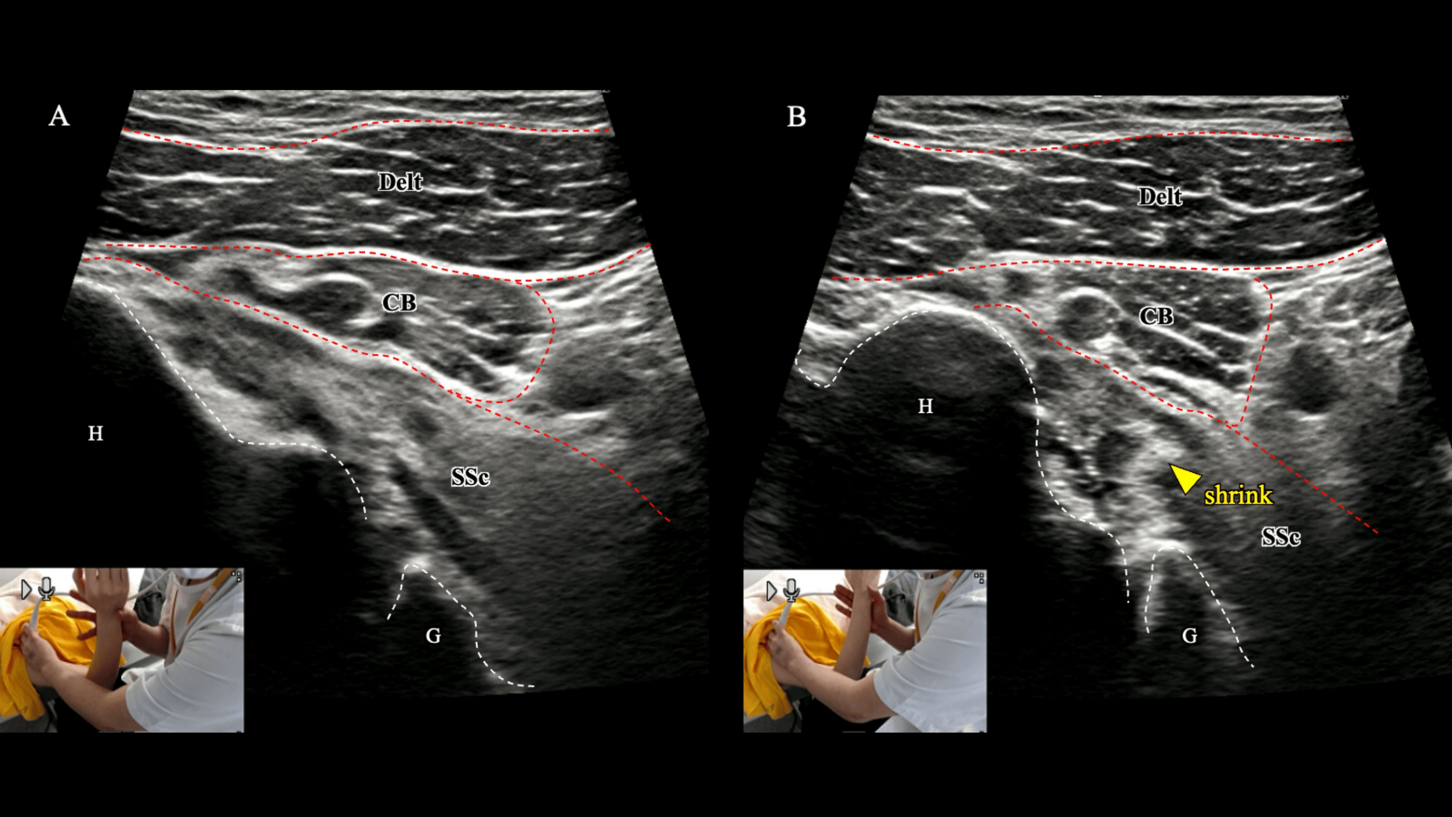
Ultrasonographic assessment of subscapularis (SSc) dynamics during internal rotation (IR). During active-assisted IR from (A) to (B), the SSc shows anterior tissue folding, incomplete humeral-head translation at end range, and compensatory activation of other internal rotators, consistent with the patient’s perceived weakness. Delt, deltoid; CB, coracobrachialis; G, glenoid; H, humeral head.
